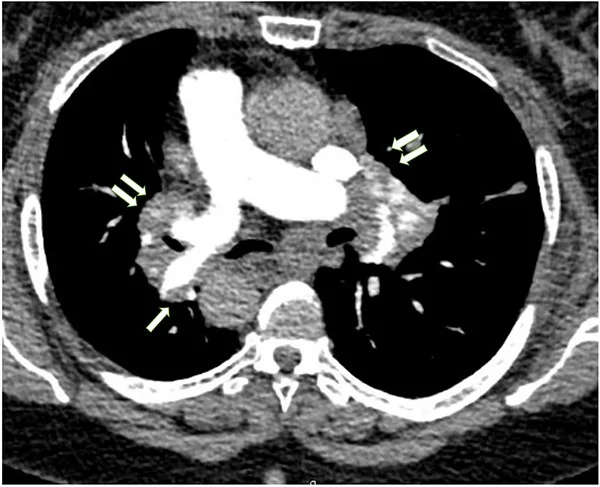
CT pulmonary angiography. Contrast-enhanced CT pulmonary angiography demonstrating bilateral hilar and mediastinal lymphadenopathy (paired arrows) and a right lower lobe pulmonary arterial filling defect (single arrow) consistent with acute pulmonary embolism.
